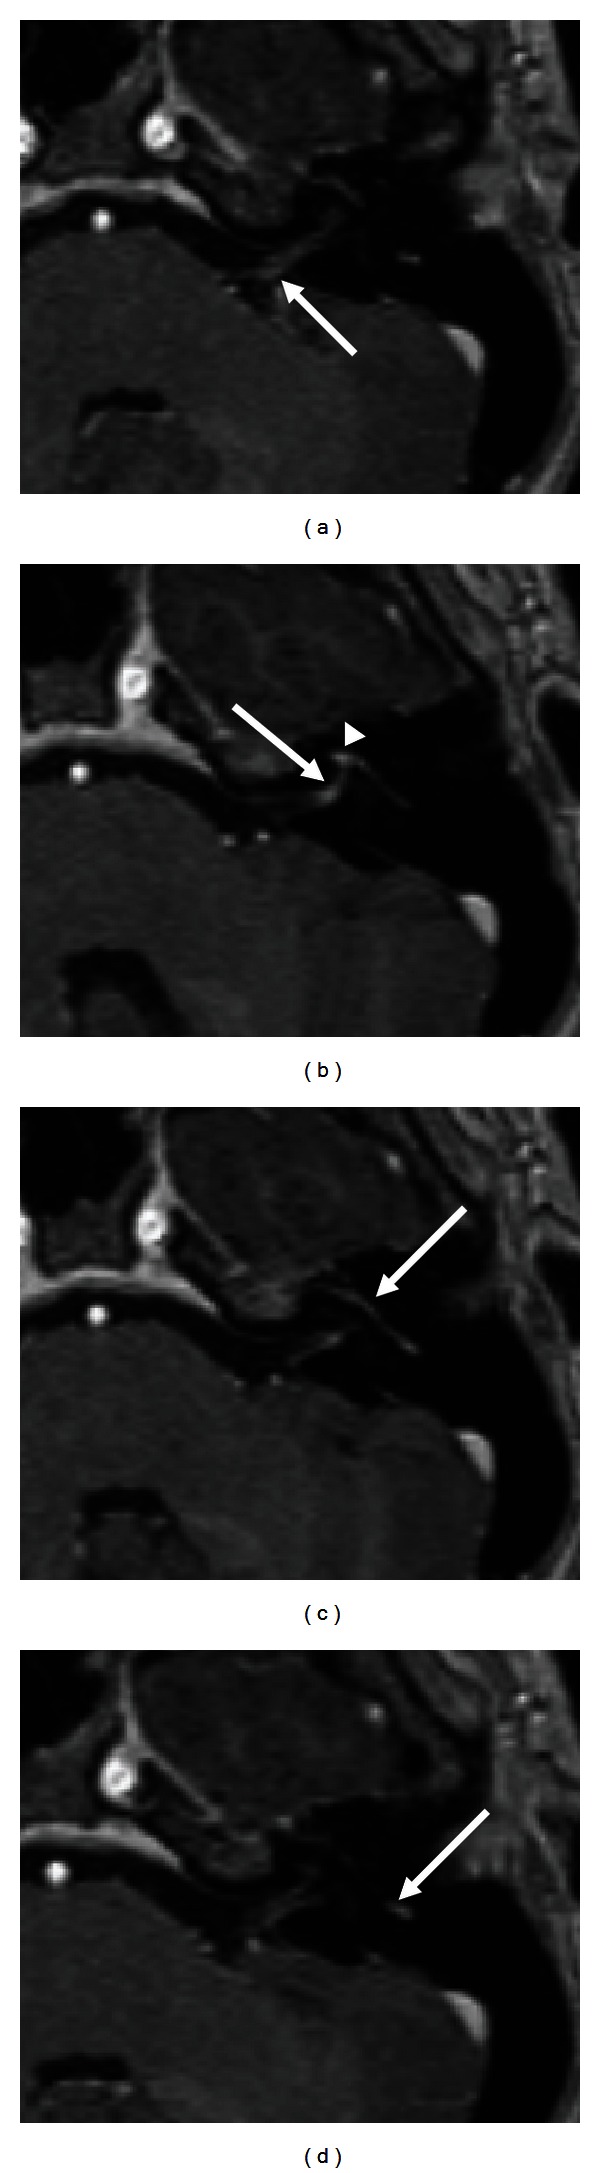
Bell's palsy. T1- weighted contrast-enhanced MR images demonstrate abnormal enhancement of the distal left cisternal (arrow, (a)), labyrinthine (arrow, (b)), first genu (arrowhead, (b)), and mastoid (arrow, (d)) segment of the facial nerve. Enhancement of the first genu, tympanic and mastoid segments was asymmetrically greater than the normal contralateral side (not shown).
